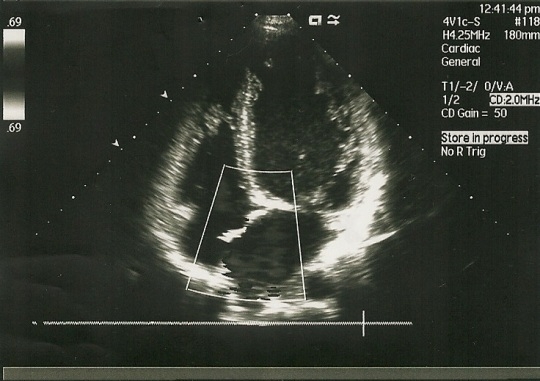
Echocardiography: LV trabeculation
